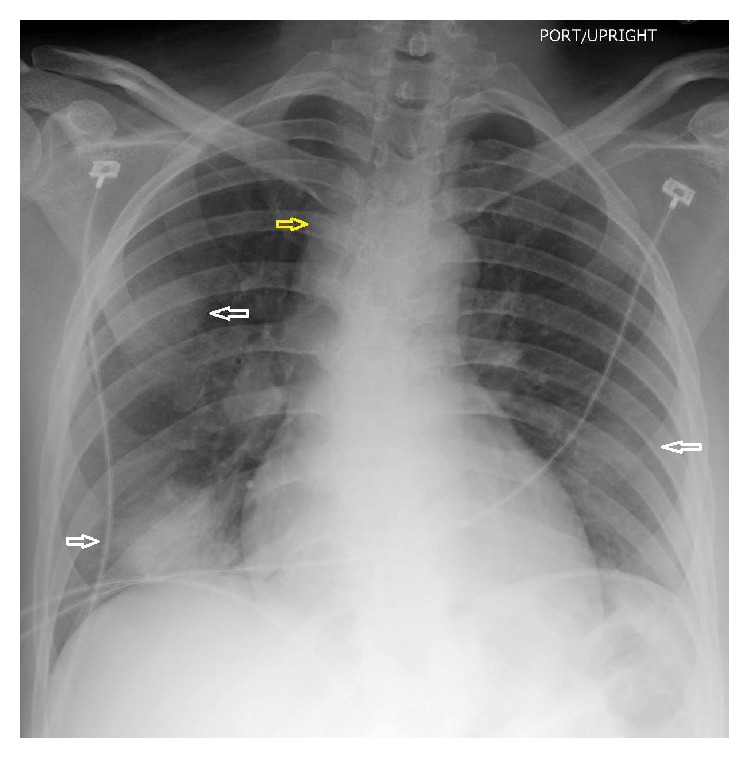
Chest X-ray: multiple bilateral pulmonary masses (white arrows) with right paratracheal lymphadenopathy (yellow arrow).
